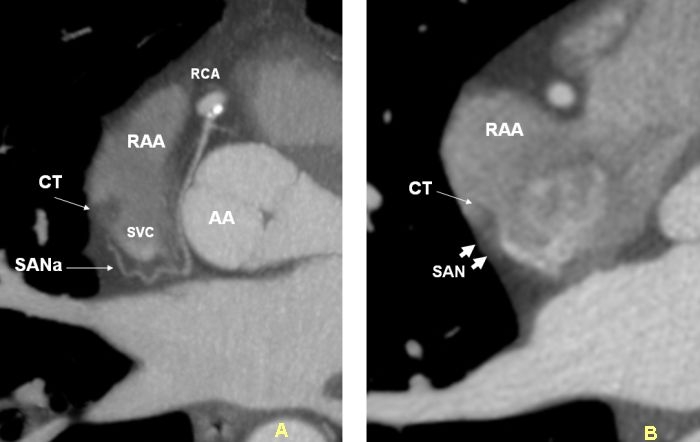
Axial images in two different patients. A. demonstrates the arterial supply of the sinoatrial node artery (SANa) arising from the proximal right coronary artery (RCA). Terminal segment of the SANa passes behind the superior vena cava (SVC) (retrocaval). Retrocaval course occurs in 47% of individuals. B. The terminal portion of the SANa is not seen. However enhancing SA node (SAN) (small arrows) can be seen in epicardial aspect of the crista terminalis (CT). CT is partially infiltrated by fat. AA=ascending aorta.
